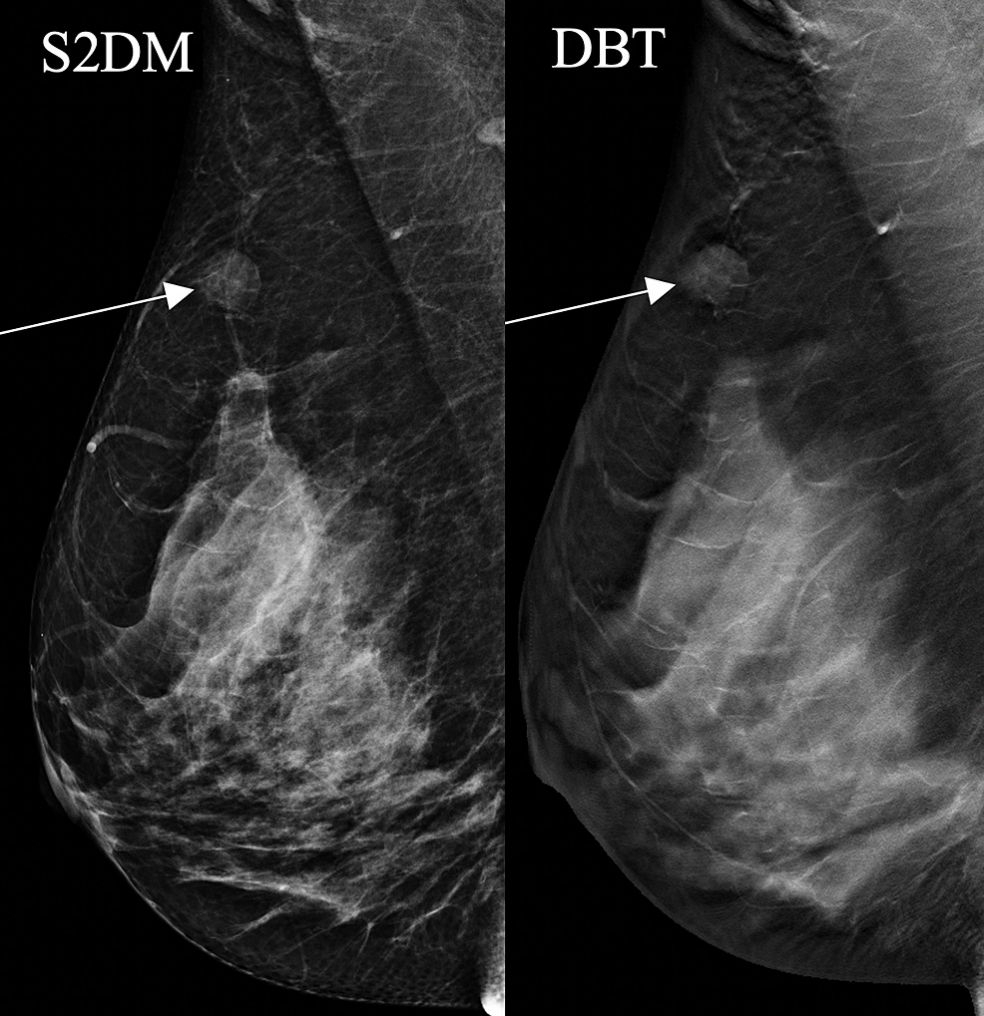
A 36-year-old woman presented with a right breast lump. Mammography demonstrated a corresponding lesion in the skin. Scrolling to the lateral most image slice on DBT confirms that the lesion is in the skin (arrows). Ultrasound demonstrated a partly intradermal lesion which likely represents an epidermal inclusion cyst. SM: synthesized mammography; S2DM: standard two-dimensional digital mammography; DBT: digital breast tomosynthesis
